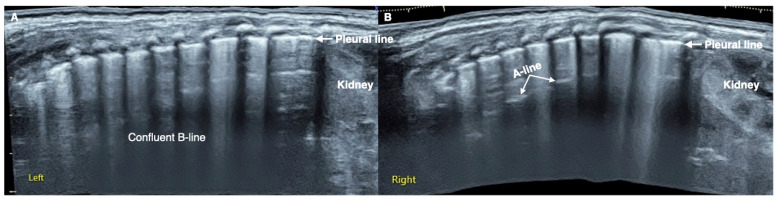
The convalescent ultrasound findings of this L. pneumoniae. LUS (extended view) showed significant confluent B-lines in both lung fields ((A): left lung, (B): right lung), the pleural line is rough and fuzzy, A-line is present only in a few intercostal spaces of the right lung (B). Lung consolidation and atelectasis disappeared in the whole lung fields. It is suggested that there is obvious pulmonary edema in the infant’s lungs.
